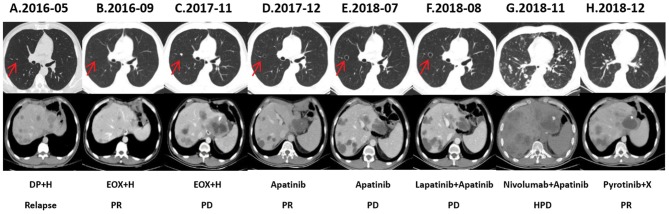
Chest and abdominal computed tomography (CT) scans. (A) CT (2016-05) demonstrates multiple liver and lung metastases before first-line chemotherapy (DP: docetaxel and cisplatin) combined with trastuzumab (H). (B) Liver and lung metastases are reduced in size after second-line chemotherapy (EOX: epirubicin, oxaliplatin, and capecitabine) combined with trastuzumab. (C) CT (2017-11) demonstrates progressive disease (PD) in the lung and liver metastases when chemotherapy combined with trastuzumab failed. (D) CT (2017-12) reveals that a cavity is formed inside the pulmonary metastatic lesions, and the size of the liver metastases are reduced than before, after using fourth-line therapy (apatinib) for 1 month. (E) Lung and liver metastases are stable during apatinib treatment until 2018-07, when all the lesions progressed significantly. (F) After 4 weeks of oral lapatinib and apatinib treatment as fifth-line therapy, CT (2018-08) scans suggest new intrapulmonary nodules and intrahepatic metastases. (G) CT scans reveal hyper-progressive disease (HPD) and that the lesions are increased and enlarged significantly after treatment with nivolumab plus apatinib for 4 weeks. (H) CT scans (2018-12) show partial response (PR) in the hepatic and pulmonary metastatic lesions after using pyrotinib plus capecitabine for 1 month.
